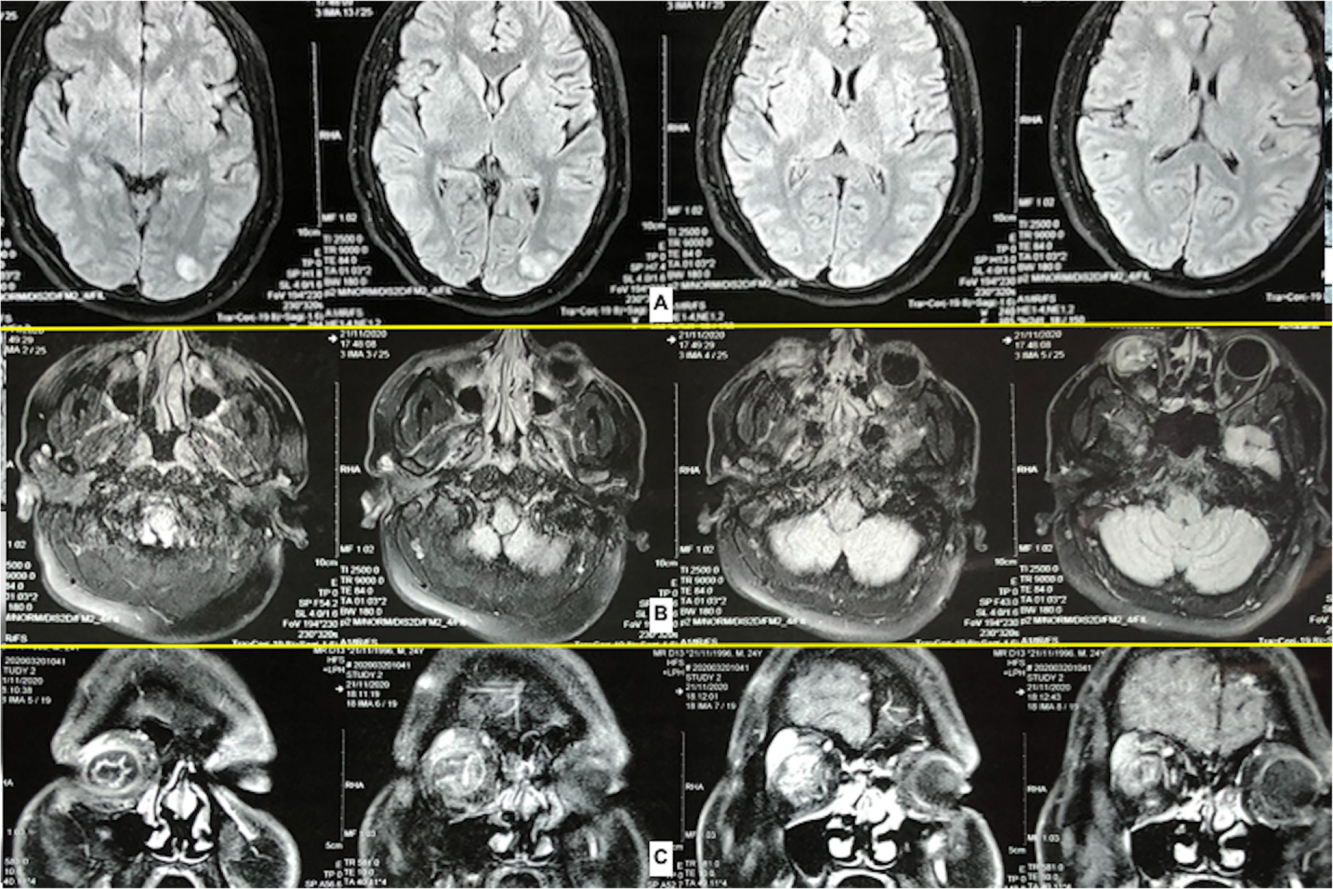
Pre-operative MRI—A multiple acute embolic infarcts in the bilateral cerebral hemisphere, B sagittal section showing the right globe appears small and distorted with T2 isointense subretinal contents along with thickened retinal and choroidal layers and C coronal section showing the same
